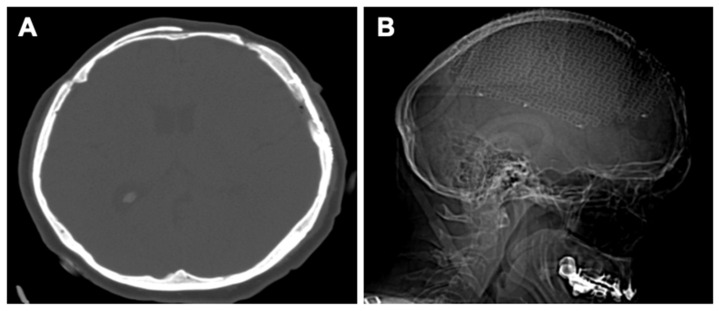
Postoperative neuroimaging: (A) Axial CT scan. (B) Sagittal radiographic scan.
